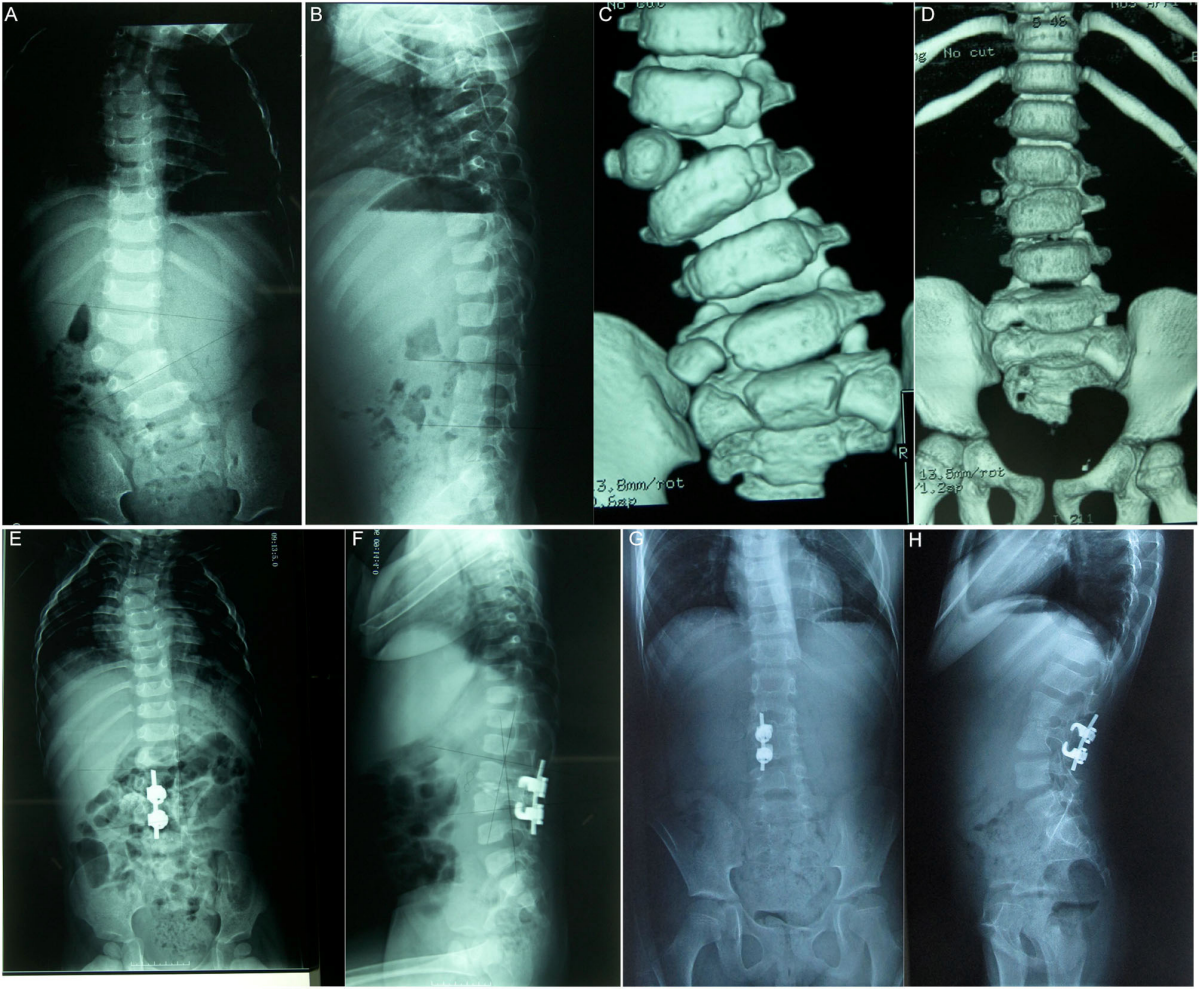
The patient is a 20-month-old boy. The right L3 is a completely segmented hemivertebrae, and the preoperative Cobb angle of scoliosis is 35°. Hemivertebra resection *via* the posterior approach of grade 5 osteotomy assisted by unilateral hook rod internal fixation was performed (A–C). Post-operative CT (D) reveals that the osteotomy space is stable, and the orthopedic effect is good. The postoperative Cobb angle of scoliosis is 7°, the orthopedic effect was good 8 years after the operation, and there was no significant loss of angle at follow-up (E,G). The sagittal position reveals that preoperative kyphosis turns into physiological lordosis (B,F,H).
